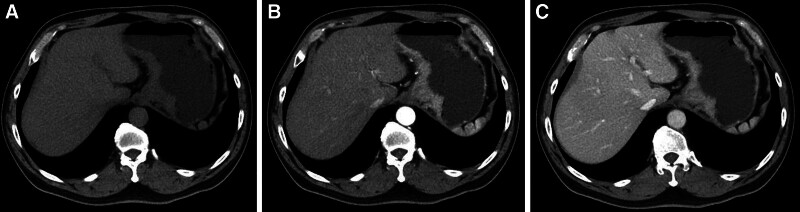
A typical case (male, 58 years old, microvascular invasion (+) and nerve invasion (+)) with local gastric wall thickening with abnormal enhancement on CT (T3 stage, N3 stage, adenocarcinoma, poor differentiated, intestinal type). (A) Plain CT image: local gastric wall thickening in the gastric cardia. (B and C) Enhanced CT image: the thickened gastric wall in the gastric cardia is significantly enhanced.
